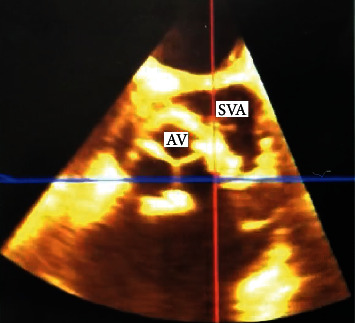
Transesophageal echocardiogram showing aortic sinus of Valsalva aneurysm extending posteriorly to interatrial septum (white arrow) and ventricular septal defect (yellow arrow). View: color Doppler, apical four chamber.
